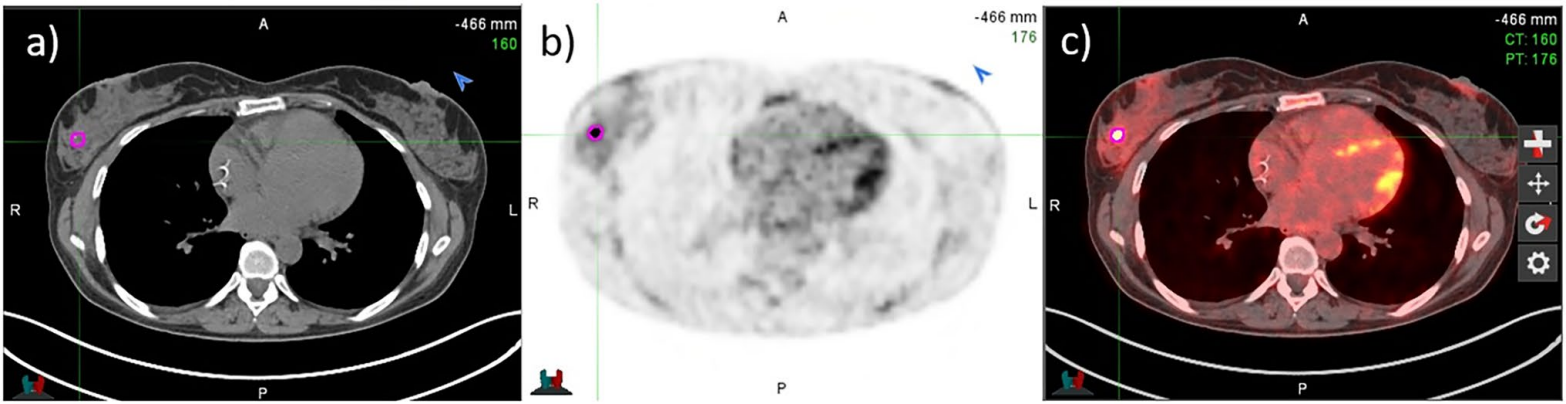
Manual segmentation of the VOI on transaxial CT (a), PET (b) and fused (c) images around the area of focal ^18^F-FDG uptake using MIM Maestro software
